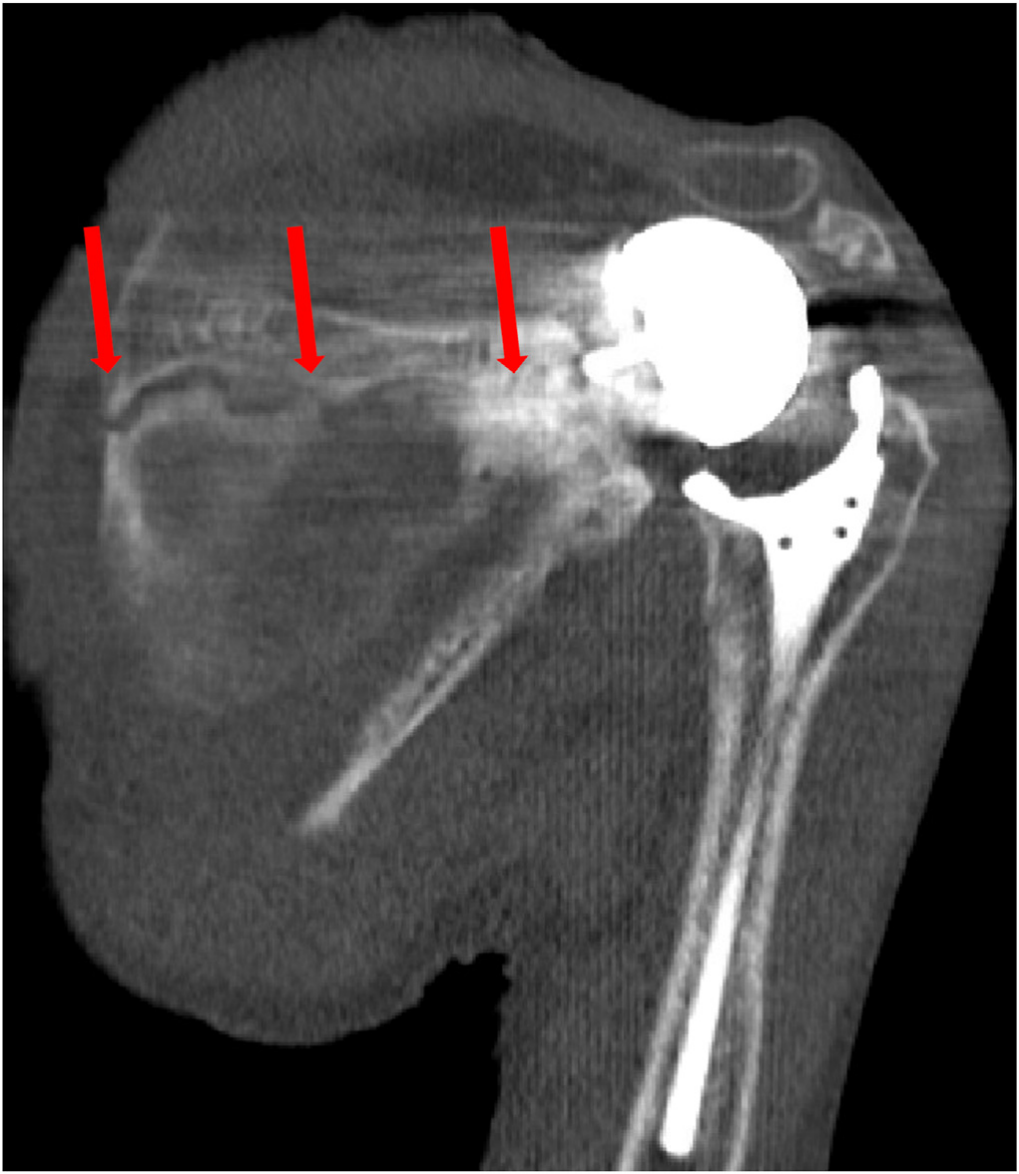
CT scan showing peri-implant scapular fracture with signs of glenoid loosening. *CT*, computed tomography.
